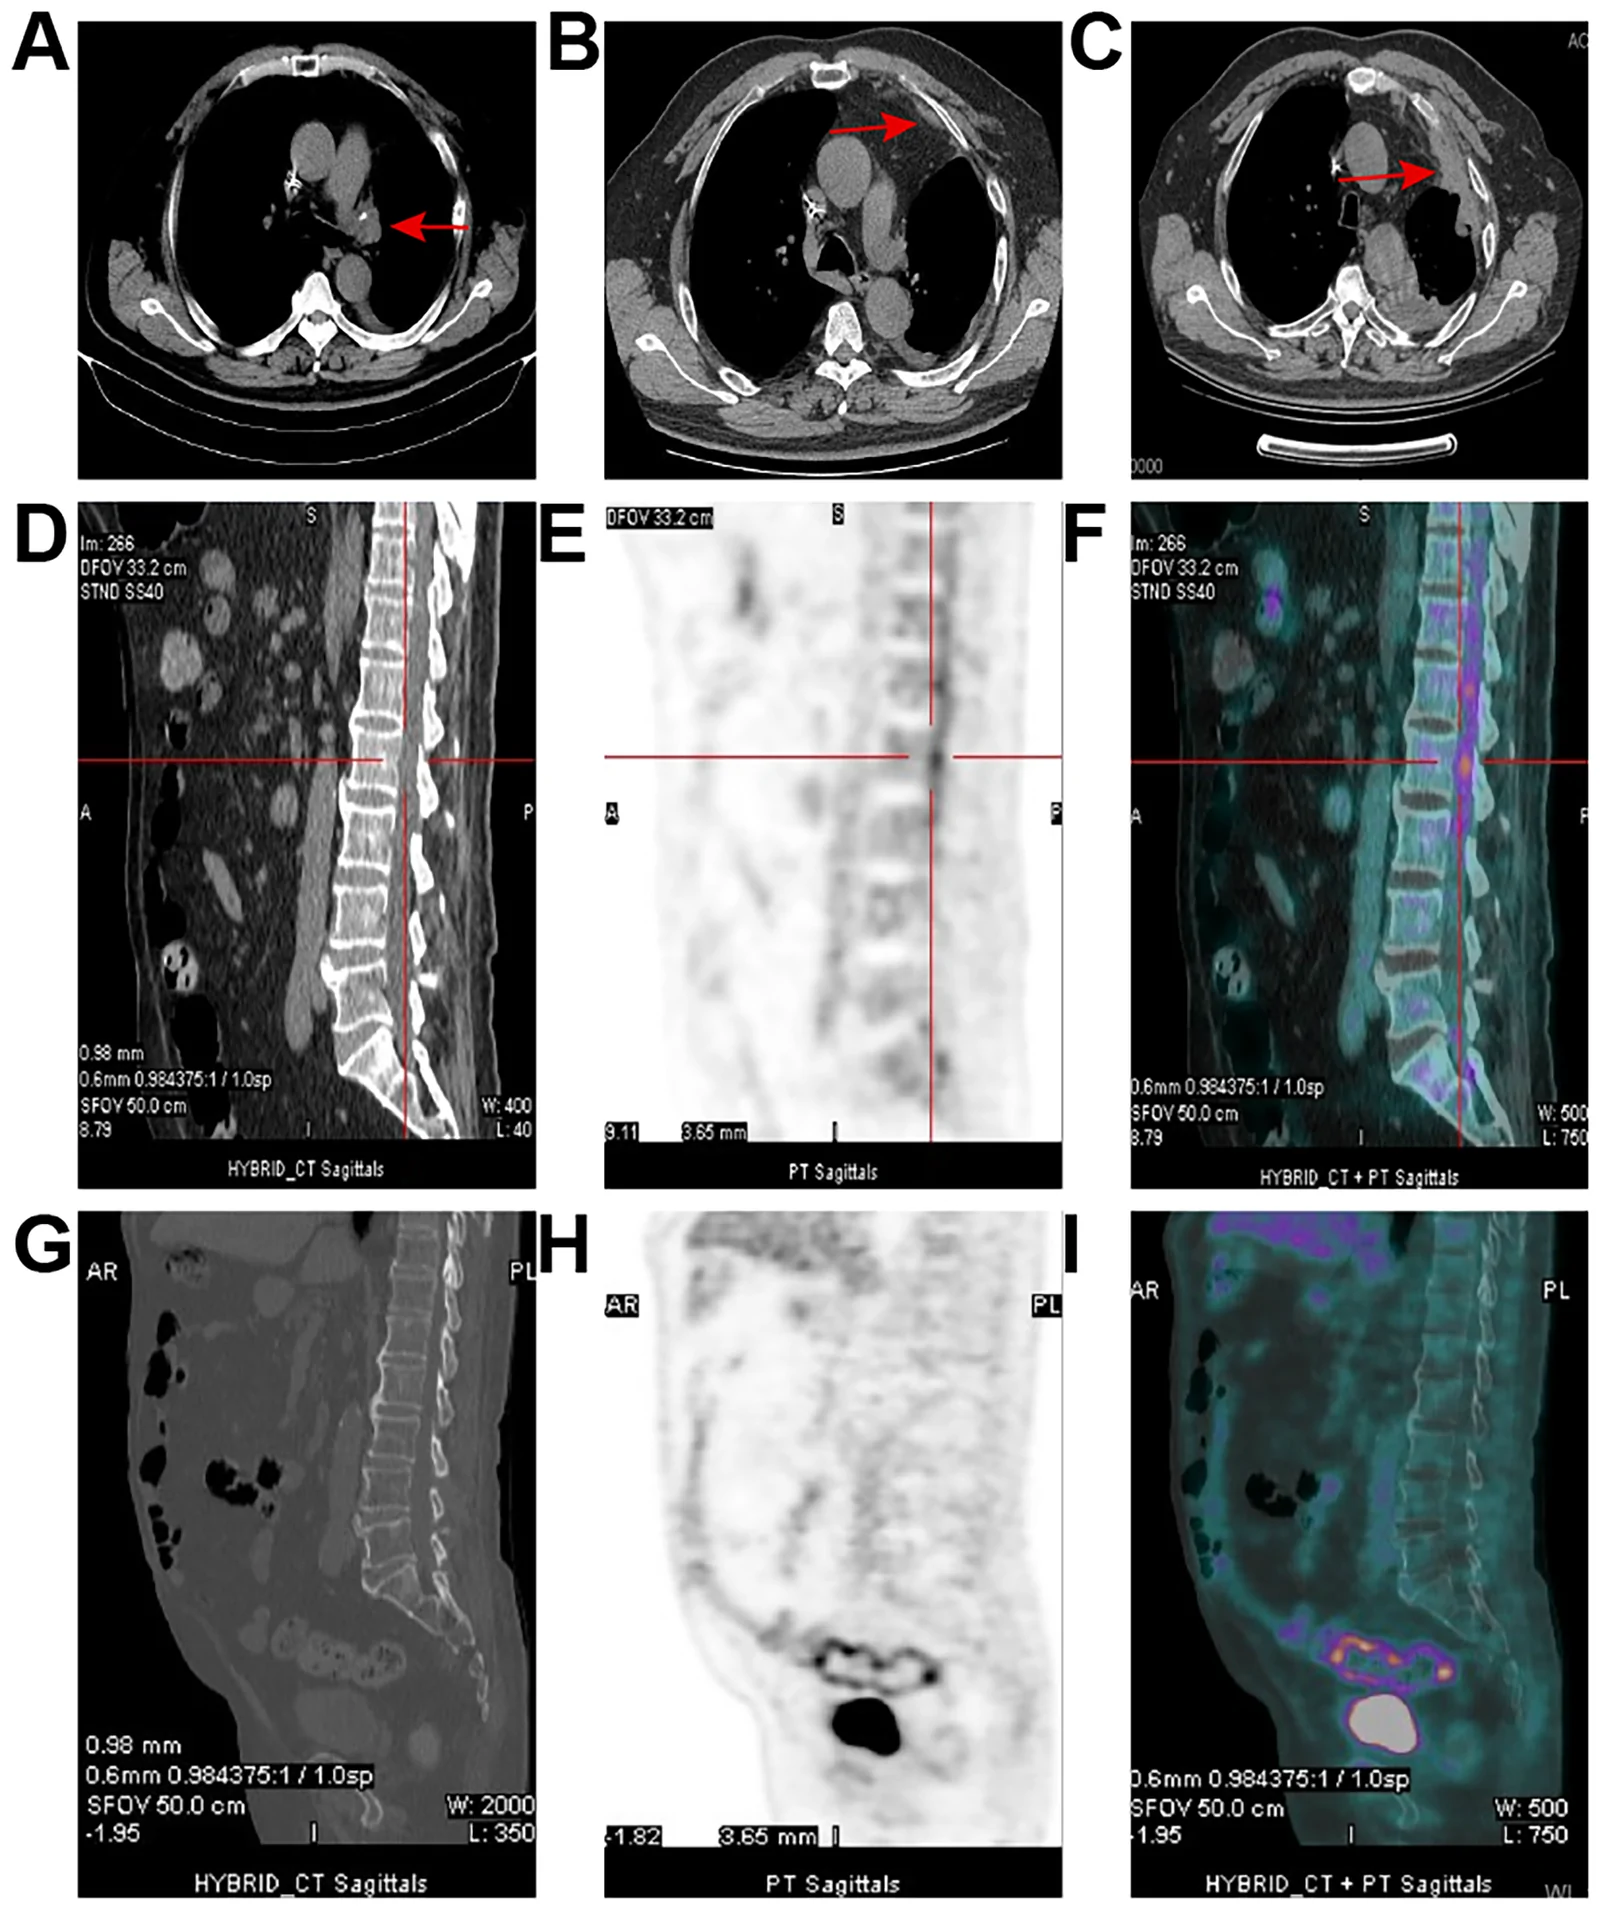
Images of diagnostic imaging results. CT imaging findings (A-C). A preoperative CT imaging on June 8, 2016 revealed a soft-tissue nodule measuring approximately 22 mm × 18 mm (A). A CT scan performed on March 22, 2018, demonstrated bilateral pleural thickening (B). The nodular pleural thickening involving the left hemithorax noted on April 18, 2019 (C). Findings from the PET/CT examination. (D-F) PET/CT scan performed on May 26, 2022, revealed segmental hypermetabolism with increased glucose uptake in the thoracic, lumbar, and sacral spinal canals, with a SUVmax of 7.16, raising concern for newly developed metastatic disease. (G-I) PET/CT scan performed on October 31, 2025, showed that the previously increased FDG uptake in the thoracolumbosacral spinal canal observed on May 26, 2022, was no longer evident. PET/CT was utilized to evaluate treatment response.
